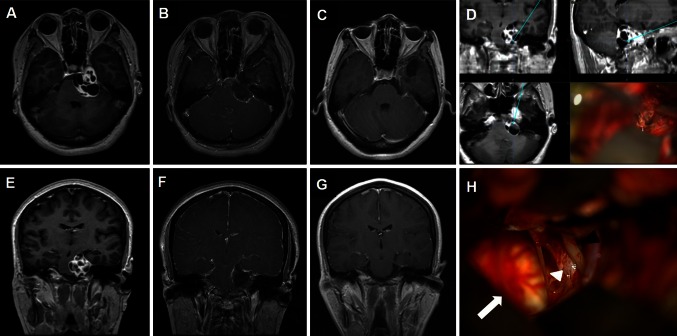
a and e Preoperative MR images of an anterior CPA schwannoma in a 46-year-old female patient. b and f Immediate postoperative MR images show that the tumor was totally removed via MLSO approach. c and g MR images show no recurrence of tumor 20 months after surgery. d The anterior CPA lesion and the tumor. h The pons (*black arrow*) was decompressed well with preservation of 3rd (*black arrow head*) and 4th (*white arrow head*) nerves after total resection of the tumor
